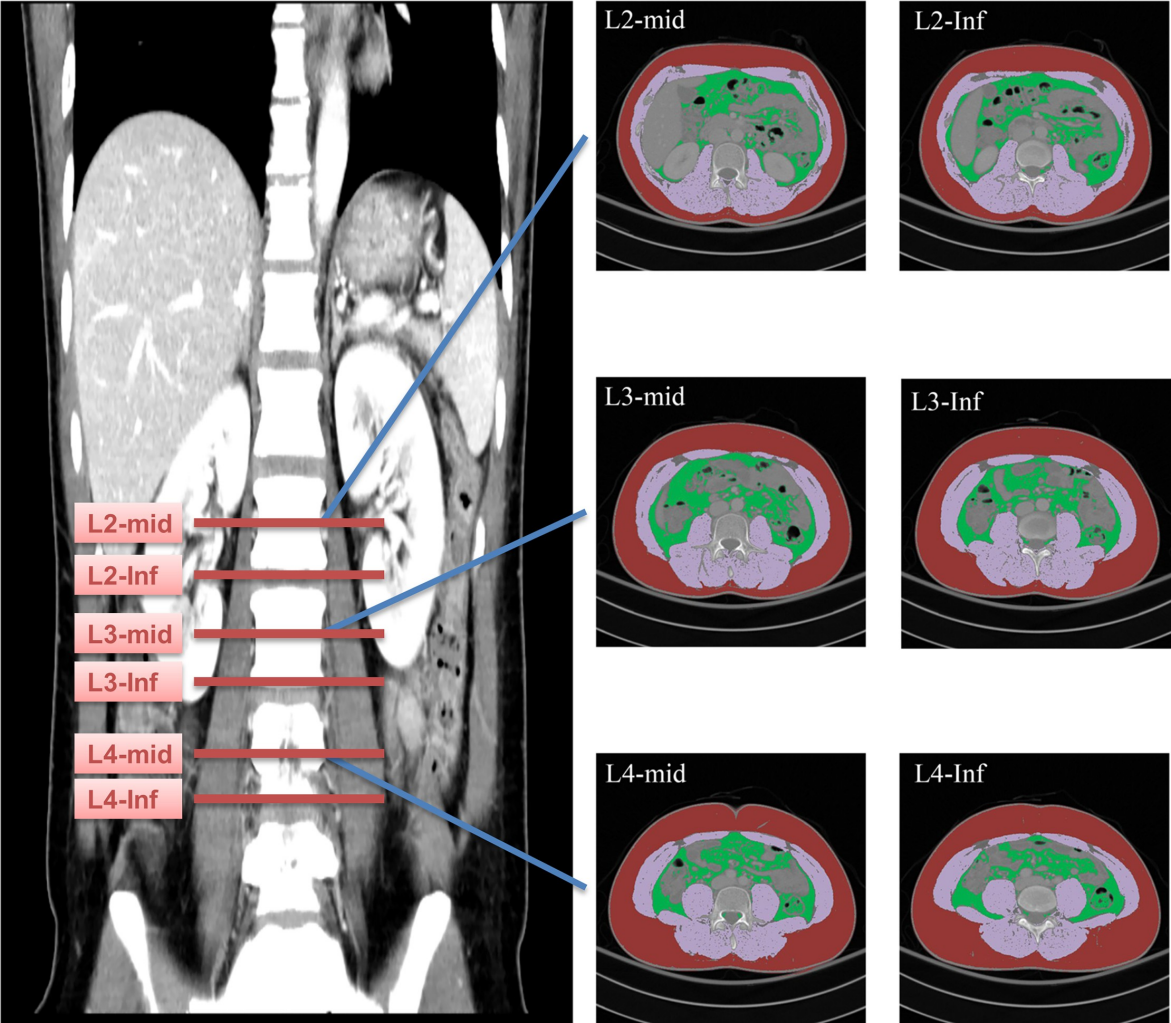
Representative images of TAMA, TPA, and VFA at different measurement levels. The vertebral bodies were measured at different vertebral levels. The axial CT images were segmented into TAMA (purple), TPA (blue), and VFA (green).
